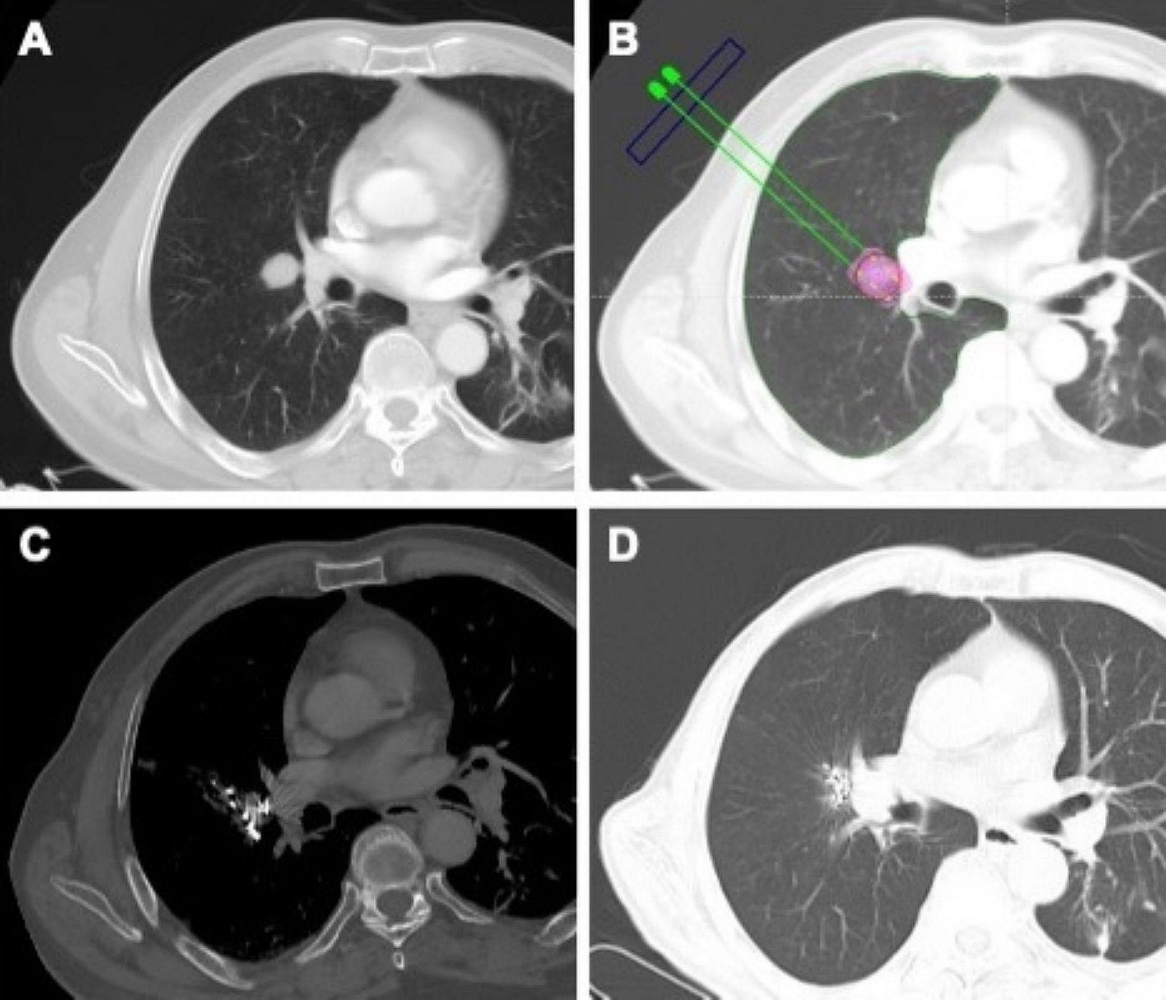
A representative case of brachytherapy. A A metastatic lesion adjacent to the right hilum (yellow arrow). B Treatment plan before brachytherapy (red region—D90 coverage area; green line—delineated organ at risk). C CT scan after brachytherapy (high-density dots—implanted iodine-125 seeds). D Follow-up CT at 16 months
